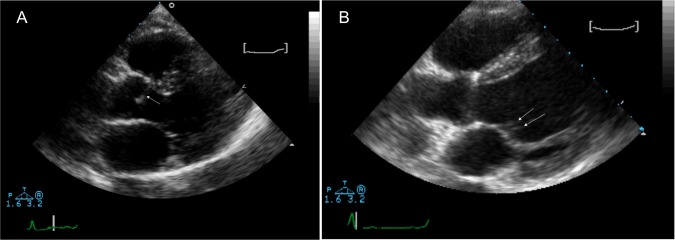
Characteristic Echocardiographic Findings. (A) Two-dimensional echocardiography image: parasternal long axis showing a thickening of the aortic sigmoid (arrows) without altering its function. (B) Parasternal long axis view of the left ventricle, showing slight thickening of the mitral leaflets (arrows), the most frequent finding in this study.
